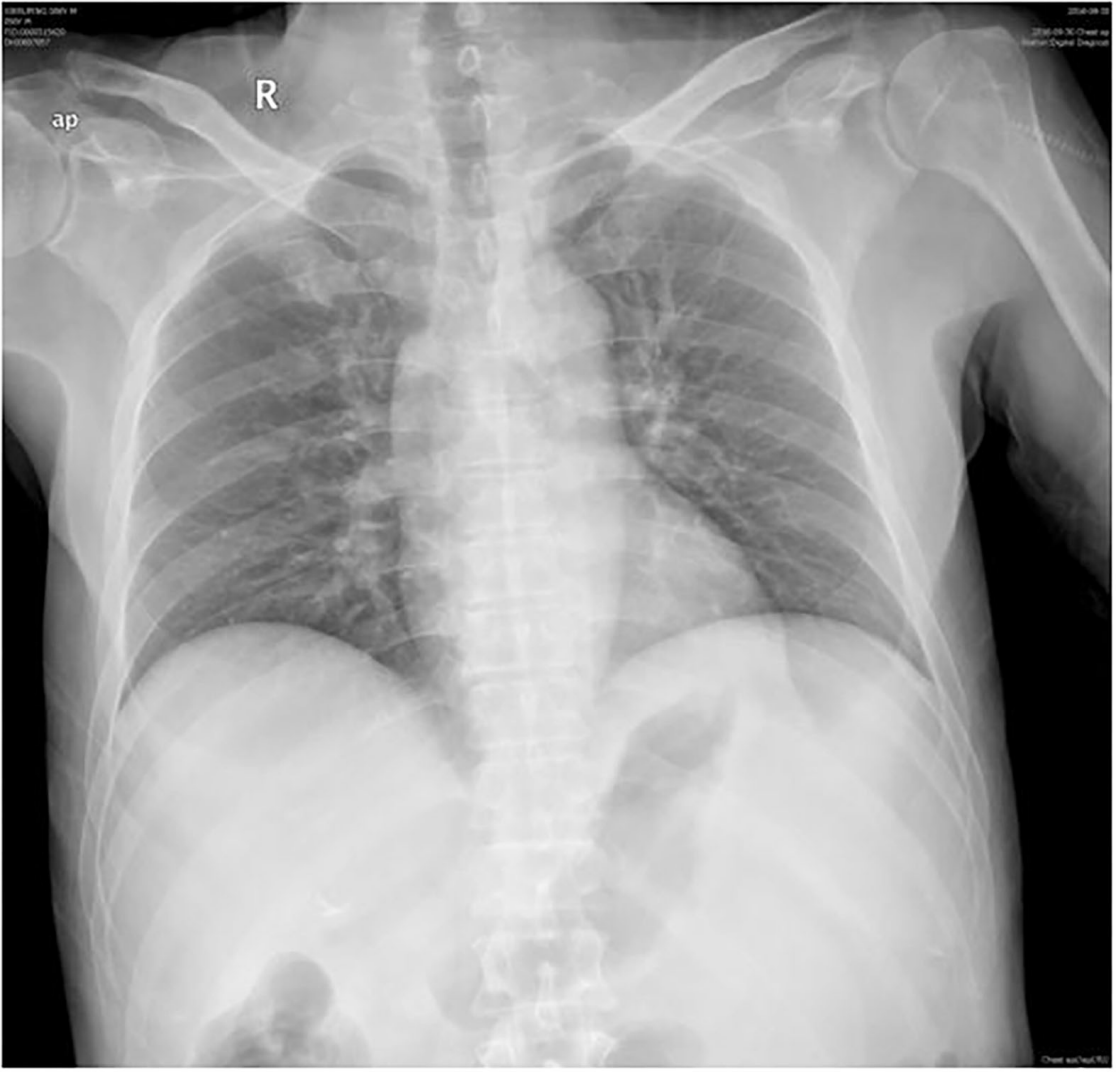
Chest radiography. The cardio-thoracic proportion of 0.47, elevating dexter diaphragm.
